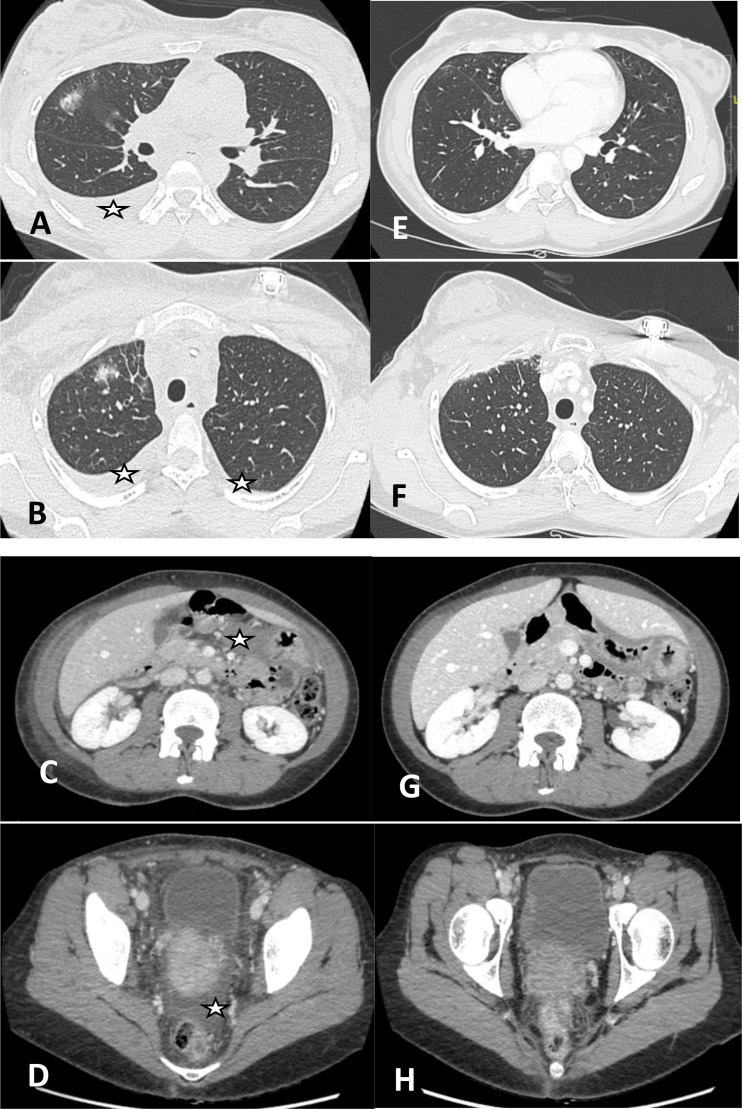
Chest and abdominal CT scans before and after corticosteroid therapy. (A, B) Bilateral pleural effusions, interlobular septal thickening, and areas of ground-glass opacity are evident prior to treatment. (C, D) Small-volume peritoneal effusion is also visible. (E–H) Follow-up scans one week after corticosteroid taper show complete resolution of pleural and peritoneal effusions and normalization of pulmonary parenchyma.
